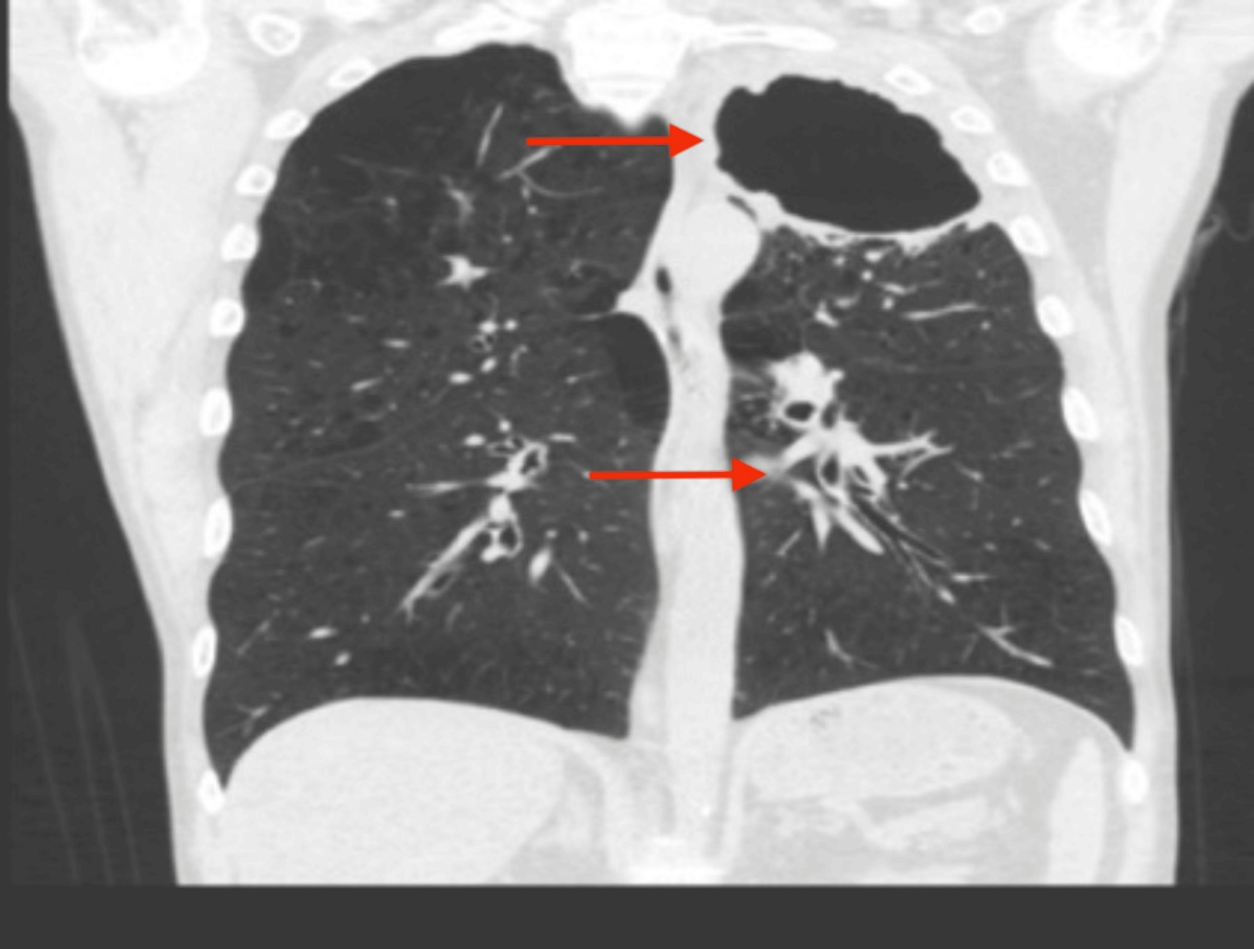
High-resolution computed tomography (HRCT) of the thorax Arrows point to evidence of disease progression, including the development of a thick-walled cavity in the left apex and multifocal solid nodules in the left upper lobe.
